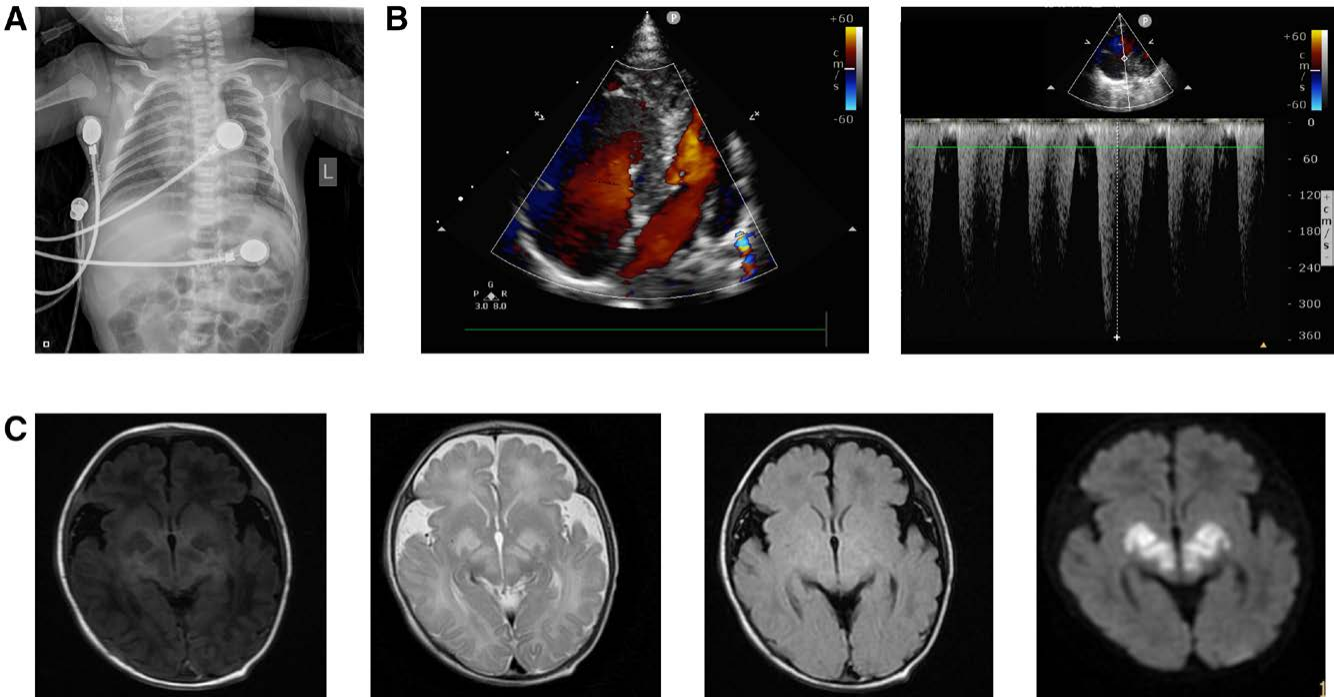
The patient suffered from neonatal pneumonia, right pulmonary hypertension, and possible leukodystrophy with CNS infection. (A) Chest CT findings. (B) UCG findings. (C) Brain MRI findings.
